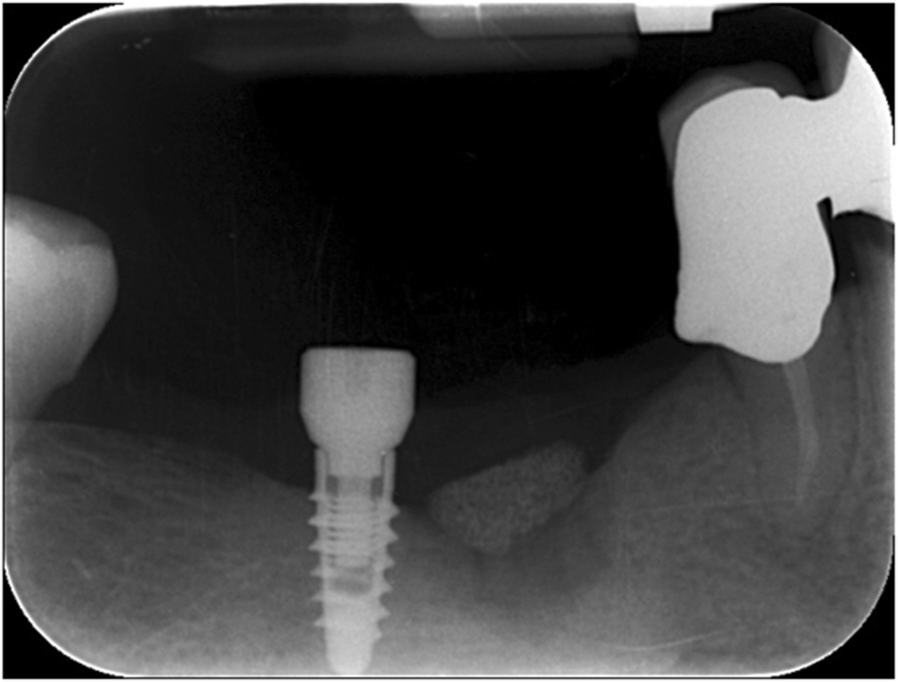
Intraoral radiography: HA scaffold placement
